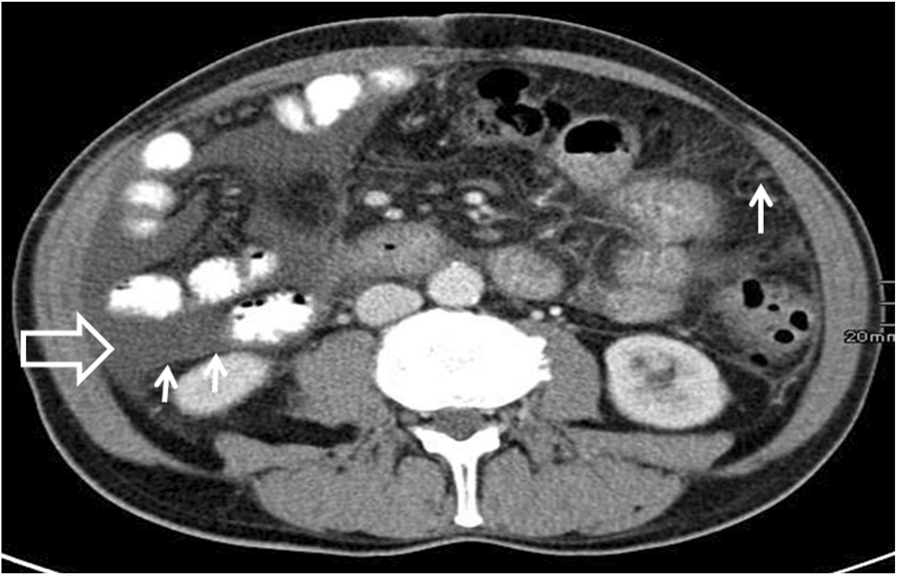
Contrast-enhanced computed tomography scan of the abdomen showing ascites (big arrow) and peritoneal nodules (small arrows) in March 2011.
